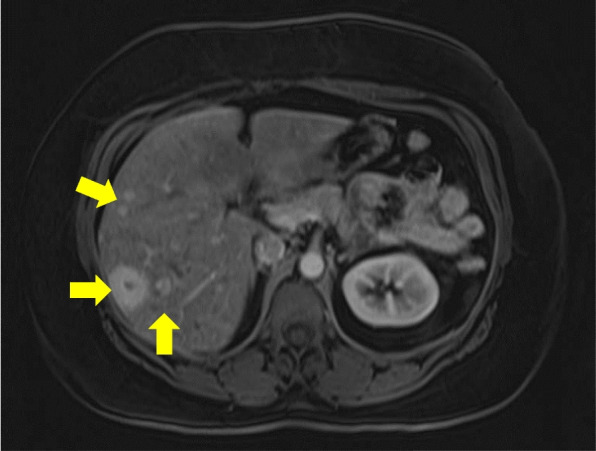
Contrast-Enhanced MRI of abdomen and pelvis. Note the innumerable mild T2 hyperintensities (see the arrows), which correlate to the arterial enhancing, rounded masses throughout the liver
